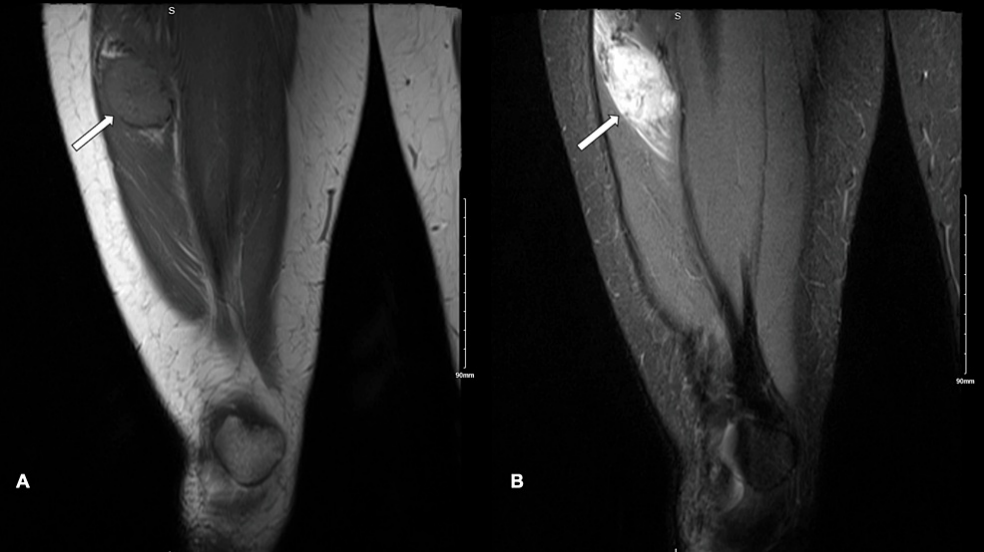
Coronal MRI of the right thigh: (A) T1; (B) T2; arrow: pointing to the lesion of interest. MRI, magnetic resonance imaging
